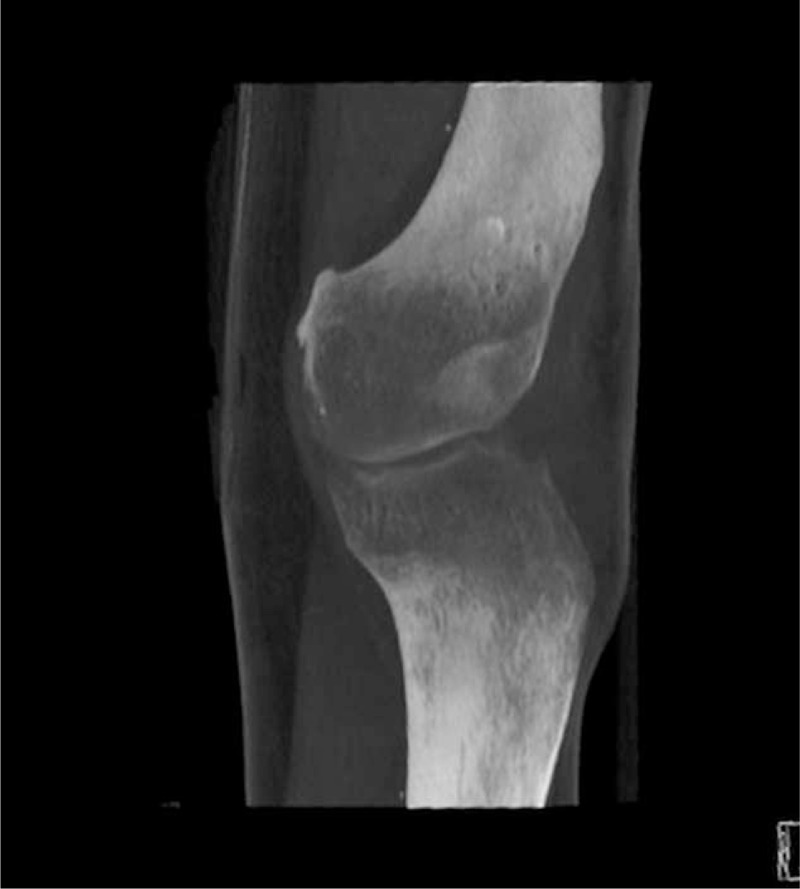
The computed tomography AP scan of the knee before arthrodesis.
